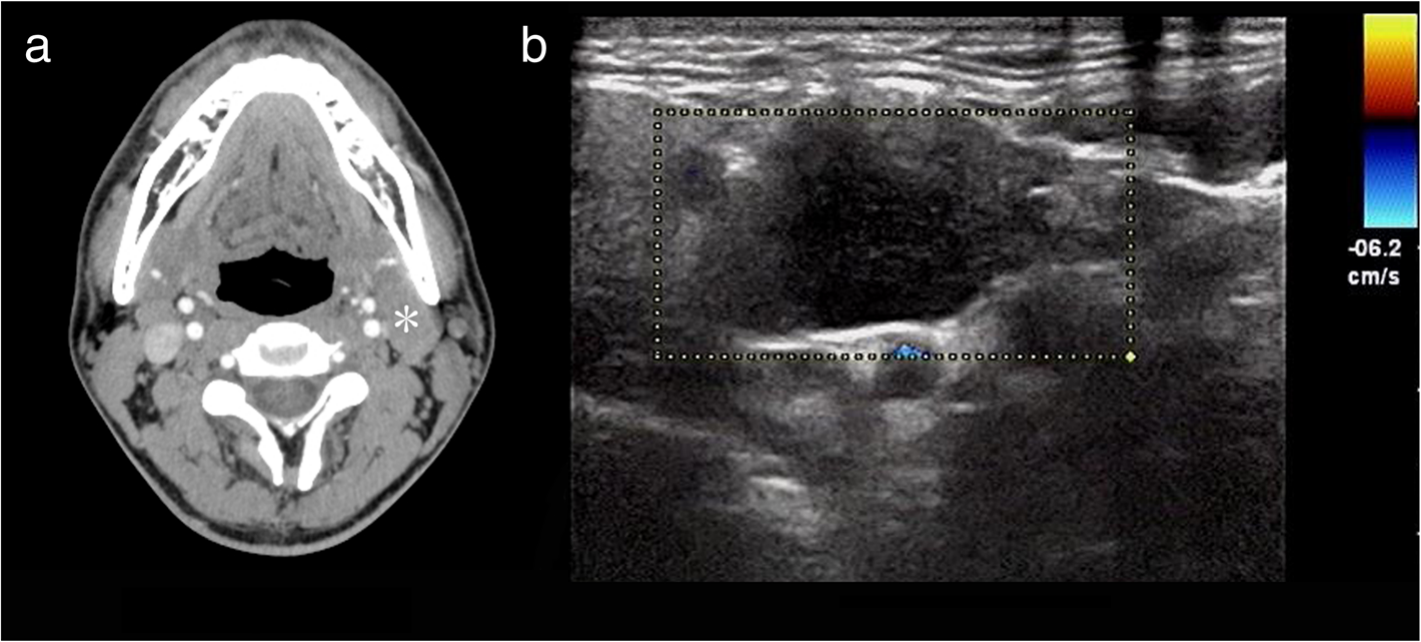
A 47-year-old woman who presented with non-Hodgkin’s lymphoma. a A contrast-enhanced CT image of the neck shows a solitary nodule (white *) with a mild enhancement pattern. b Spectral Doppler ultrasound imaging shows no blood flow
